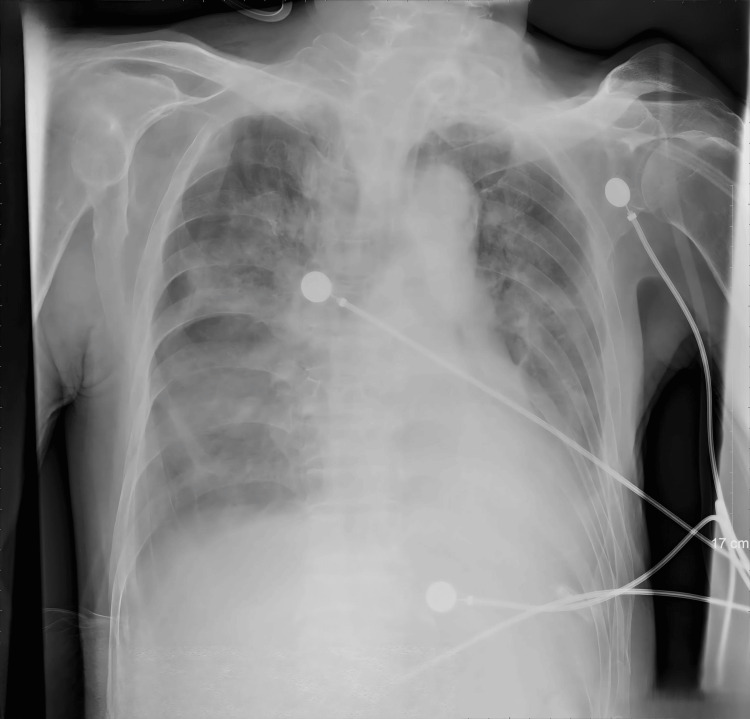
Bedside anteroposterior (AP) chest radiograph showing bilateral pneumonia with small bilateral pleural effusions
